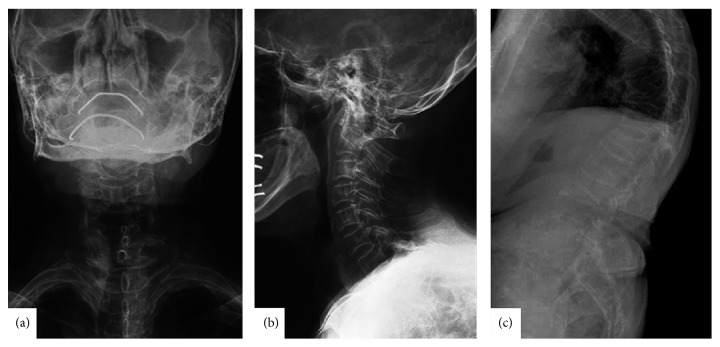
Anteroposterior (a) and lateral (b) standard radiographs of cervical spine revealed degenerative spondylosis but failed to reveal apparent fracture in cervical spine. Lateral standard radiographs of thoracolumbar spine revealed marked thoracic kyphotic deformity due to multiple compression fractures (c).
